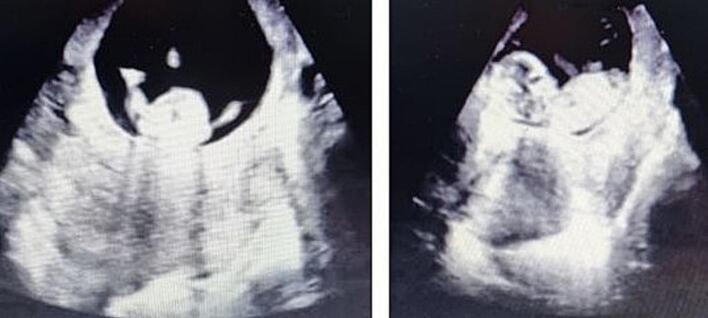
Abdominal ultrasound image of intact pregnancy with thin anterior uterine wall.
